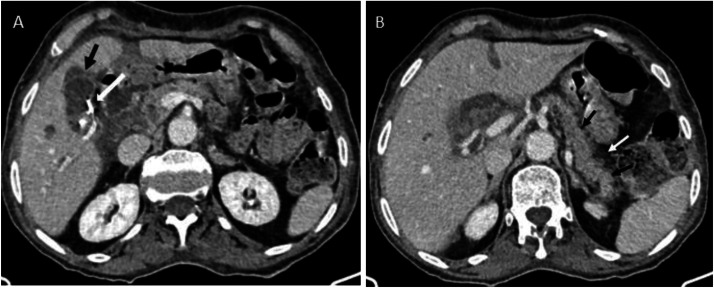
(A) Contrast enhanced computed tomography scan showing a biloma (black arrow) in the glallbladder fossa adjecent to the surgical clip (white arrow). (B) It also demonstrates a peri pancreatic fat infiltration (withe arrow) and pancreatic swelling (black arrow).
